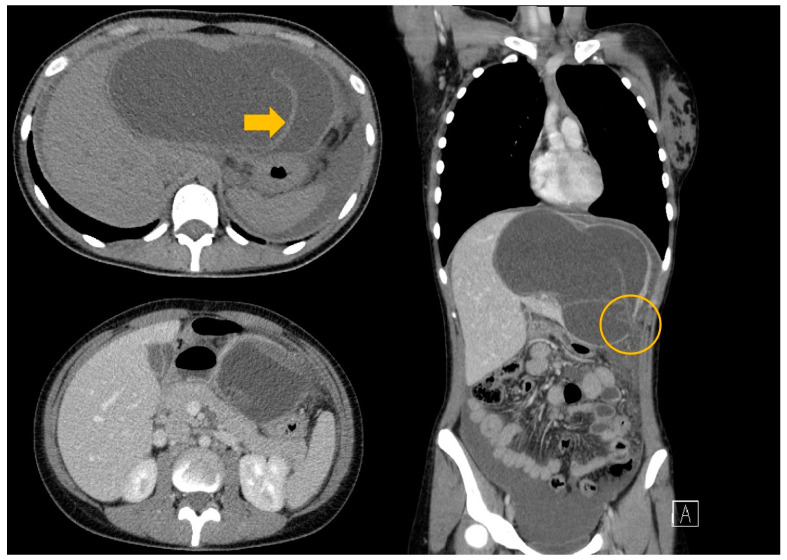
A CT scan of the thorax abdomen and pelvis with contrast medium—a giant liver hydatid cyst with rupture at the level of segment III with free peritoneal fluid (arrow—the floating internal membrane; circle—the rupture of the cyst).
